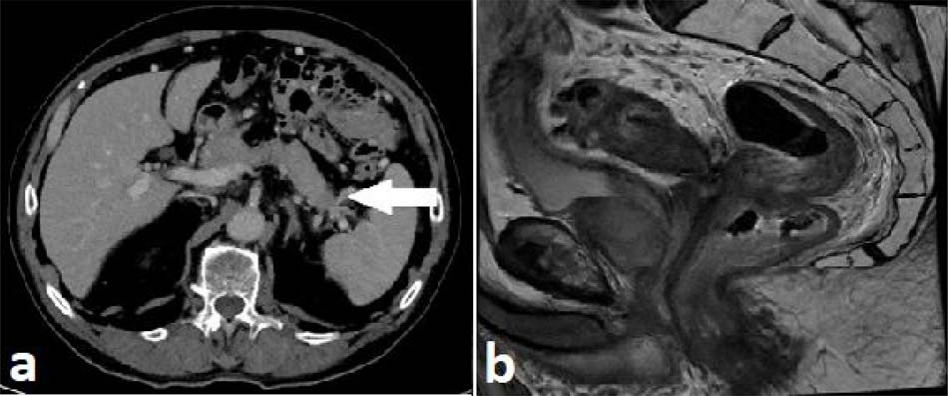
Case A. Axial portal venous phase CT scan demonstrating hypo-enhancing distal pancreatic lesion encasing splenic vessels (a) and sagittal MRI pelvis demonstrating anterior low rectal tumour (b), staged as a cT4aN2Mx rectal adenocarcinoma.
